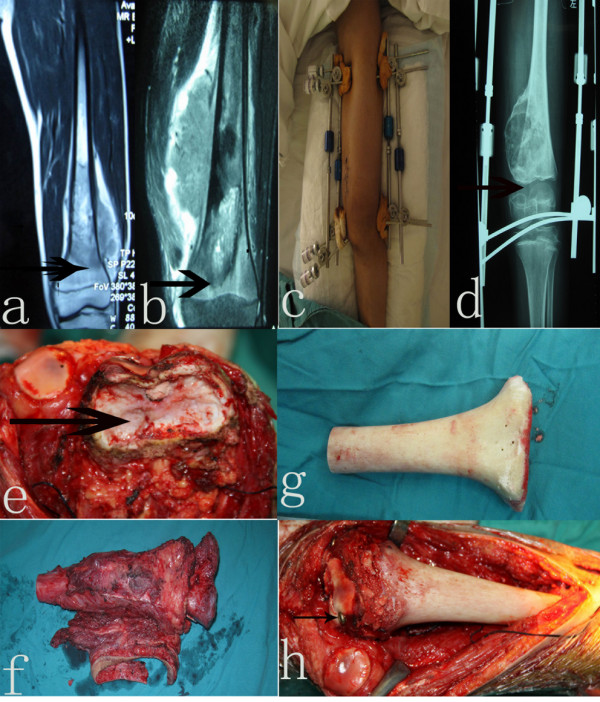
Epiphysis distraction before surgery and fixation between the epiphysis and allograft bone during surgery. a) Type I of distal femur osteosarcoma (arrow point shows the gap between osteosarcoma and the epiphyseal plate >2 cm). b) Type II of distal femur osteosarcoma (arrow point shows the gap between osteosarcoma and epiphyseal plate <2 cm). c) Gross view of epiphysis distraction by an external fixator. d) X-ray of epiphyseal distraction by an external fixator(arrow point shows the metaphyseal and epiphyseal plate were completely separated). e) Appearance of proximal epiphysis section (arrow point indicates a cross section of the proximal end of the epiphysis was irregular sawtooth shaped). f) Appearance of tumor bone after resection (the interval between osteotomy level and tumor border was no less than 5 cm). g) The distal part of the allograft bone was trimmed to be consistent with the proximal epiphysis. h) Appearance after fixation between the epiphysis and allograft bone (arrow point shows the fixation of cartilage and allograft bone by lag-screw of cancellous bone).
